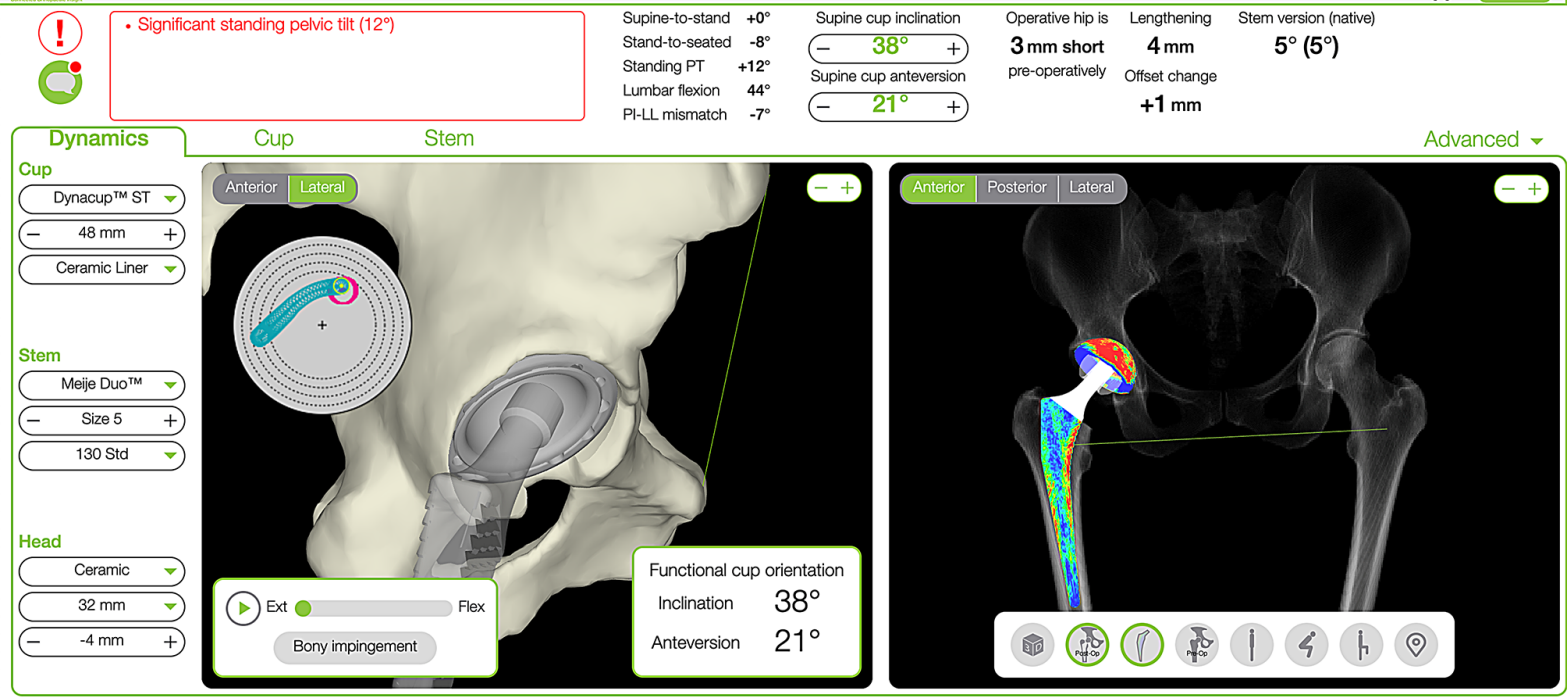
Preoperative implant positioning planning via 3D CT reconstruction. Distribution of the load sharing at the bone–implant interface (right)
